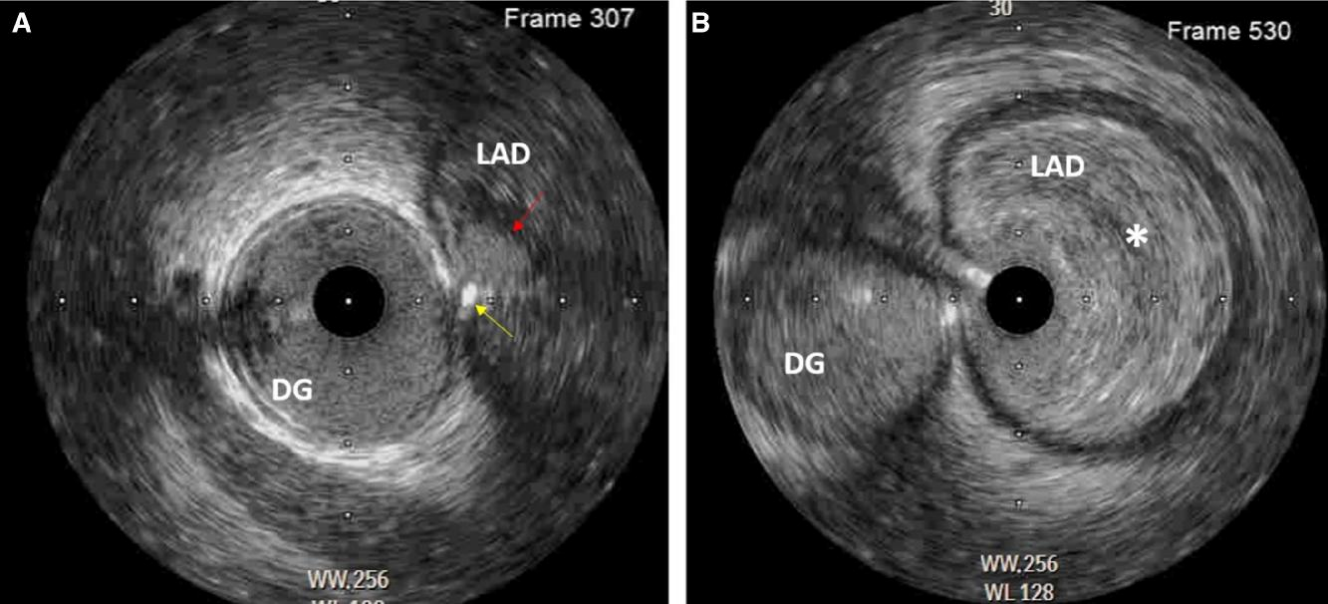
Panel (*A*) shows the intravascular ultrasound catheter at the level of the left anterior descending bifurcation, the mid-left anterior descending, and diagonal branches. The red arrow shows the small orifice of the mid-left anterior descending ostium. Using live intravascular ultrasound guided imaging, we were able to successfully wire the left anterior descending (yellow arrow). Panel (*B*) shows the intravascular ultrasound catheter within the mid-left anterior descending. Note the large burden of soft lipid rich plaque (*).
